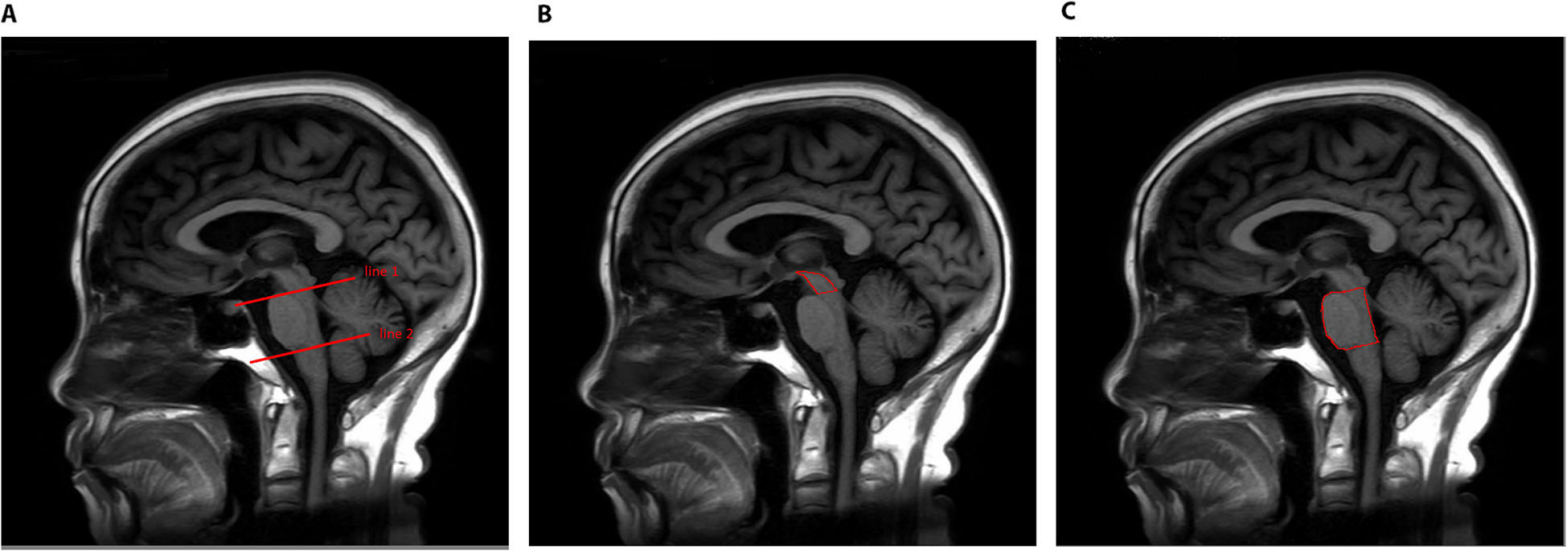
Sagittal T1-weighted MR images show midbrain area and pons area. a line 1 and line 2; b midbrain area; c pons area
